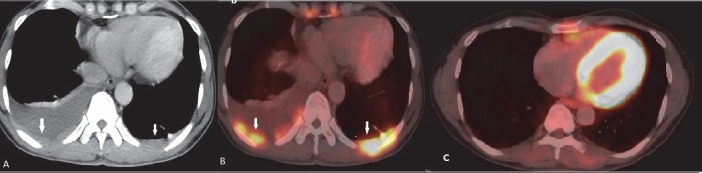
Lymphoma involving the pleura. CT scan shows enhancing pleural nodules (arrows in A) and fused PET/CT image shows increased FDG uptake (arrows in B). Complete metabolic response of the pleural lesions is seen in post chemotherapy scan. Pleural effusion has also subsided in follow up scan (C)
